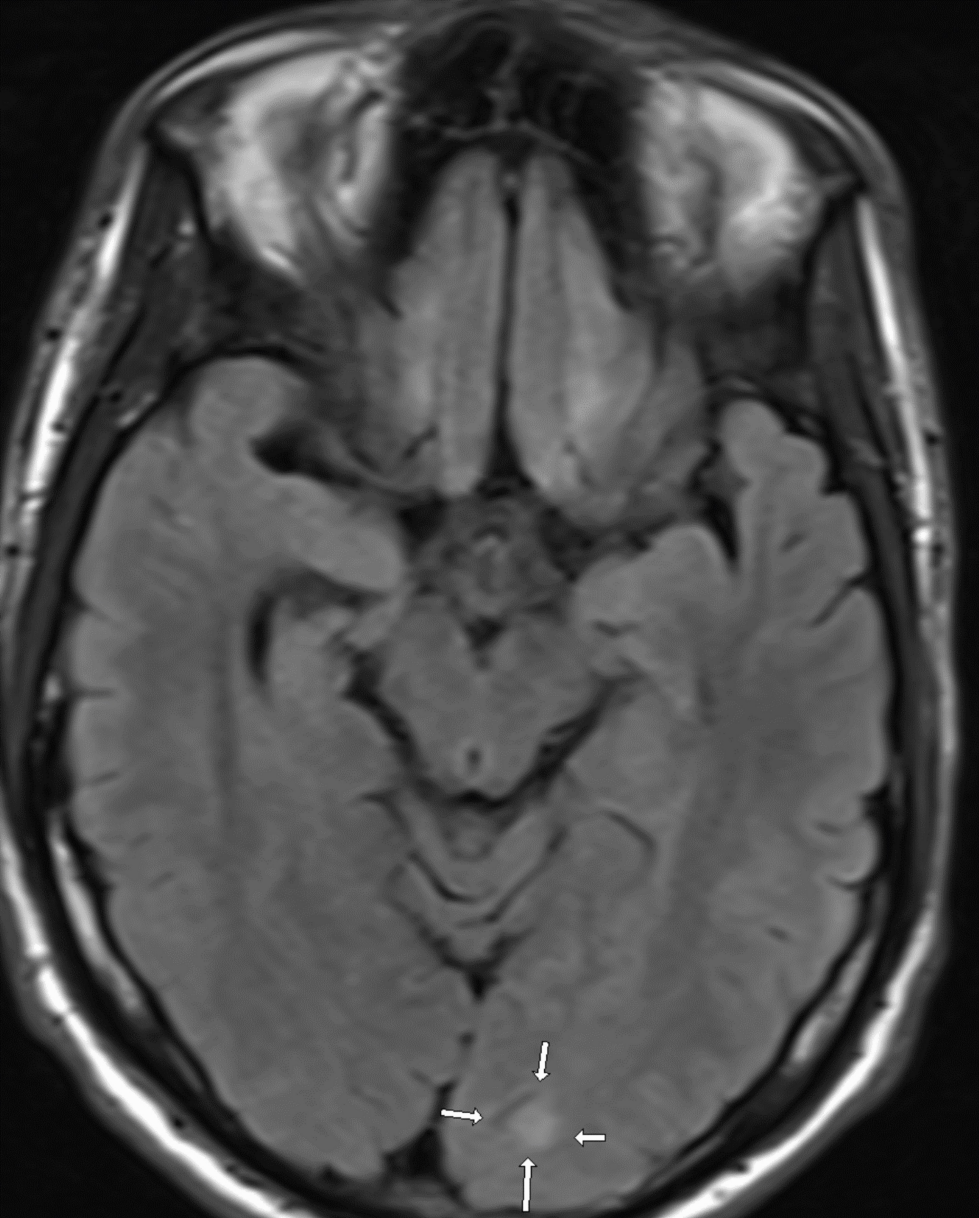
Axial MR FLAIR image of the brain showing a small hyperintense lesion at the gray-white junction in the left occipital lobe (arrows) MR: magnetic resonance, FLAIR: fluid-attenuated inversion recovery
